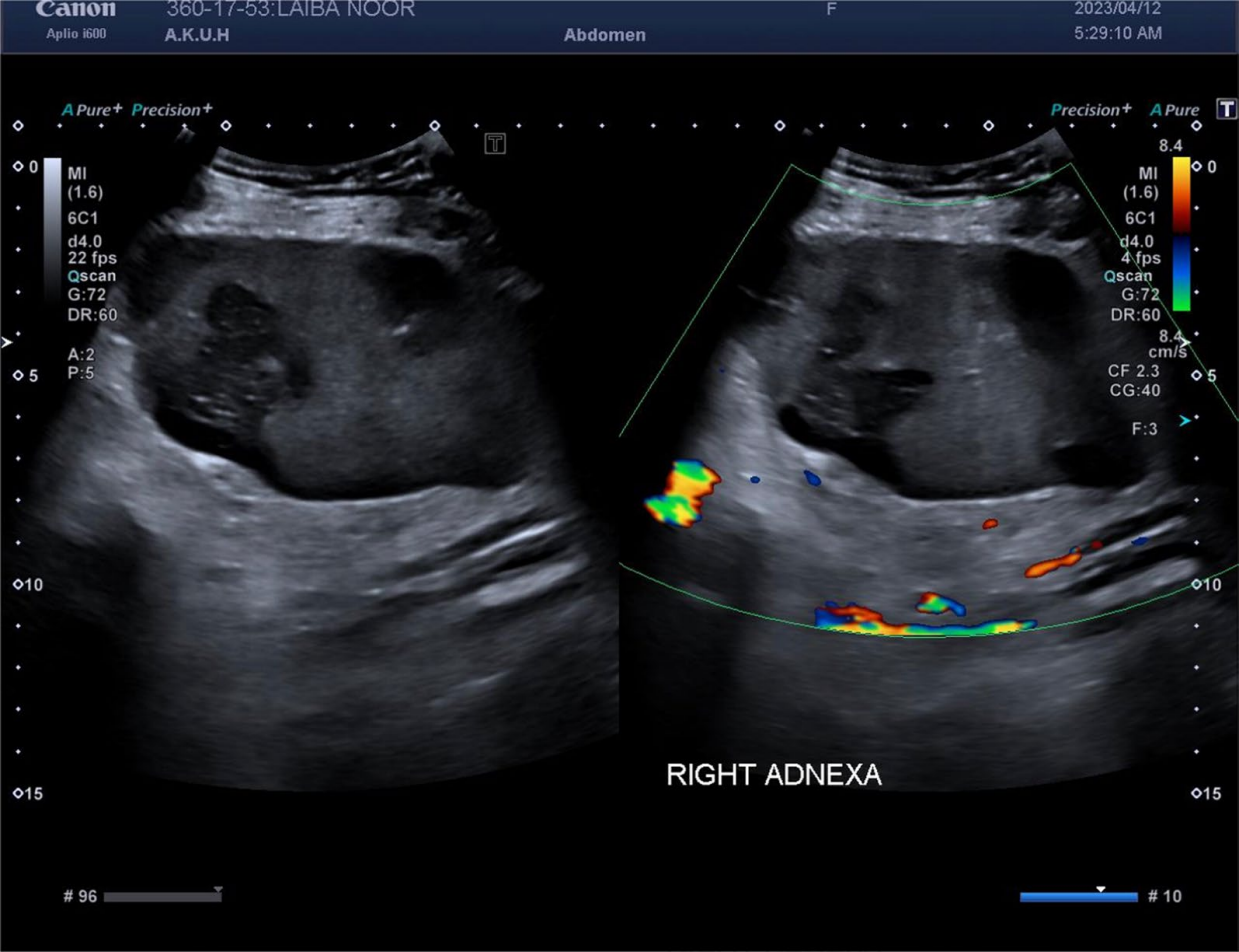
Ultrasound of right pelvis. A solid cum cystic mass with specs of calcification can be noted
